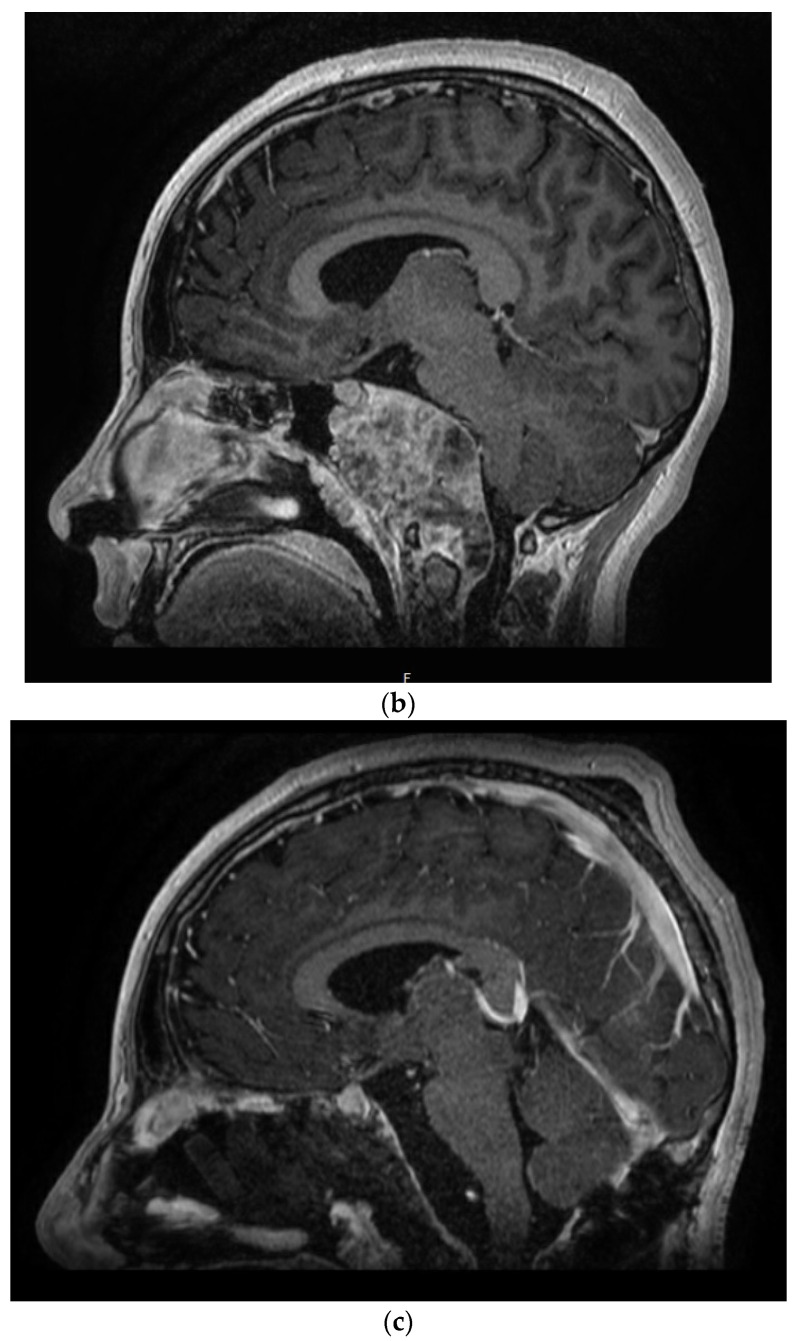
(a) T_2_-weighted MRI coronal sequence demonstrating the characteristic hallmarks of a clival chordoma, T_2_ hyperintensity within the skull base lesion. (b) T_1_-weighted MRI post-contrast sagittal sequence demonstrating anatomical boundaries of a clival chordoma with ventral brainstem compression. (c) T_1_-weighted MRI post-contrast sagittal sequence demonstrating gross total resection via endoscopic endonasal approach of clival chordoma.
